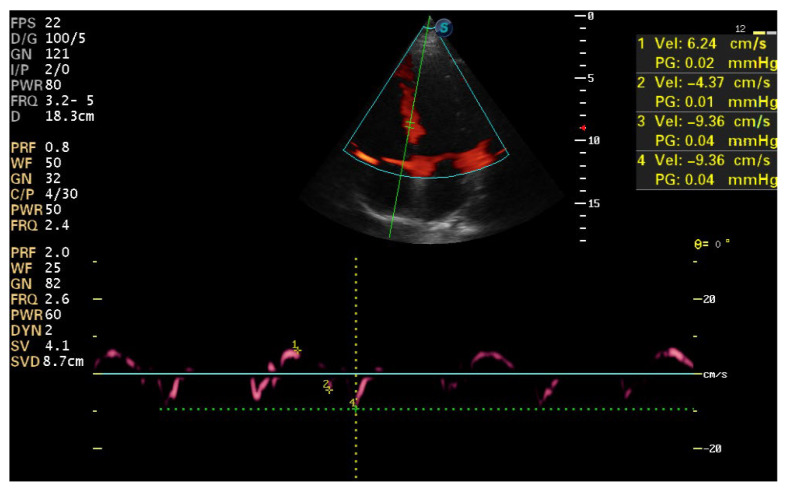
Case 1—Tissue Doppler Imaging septum evaluation (1-S wave, 2-e’ wave, 3,4-a’ wave).
